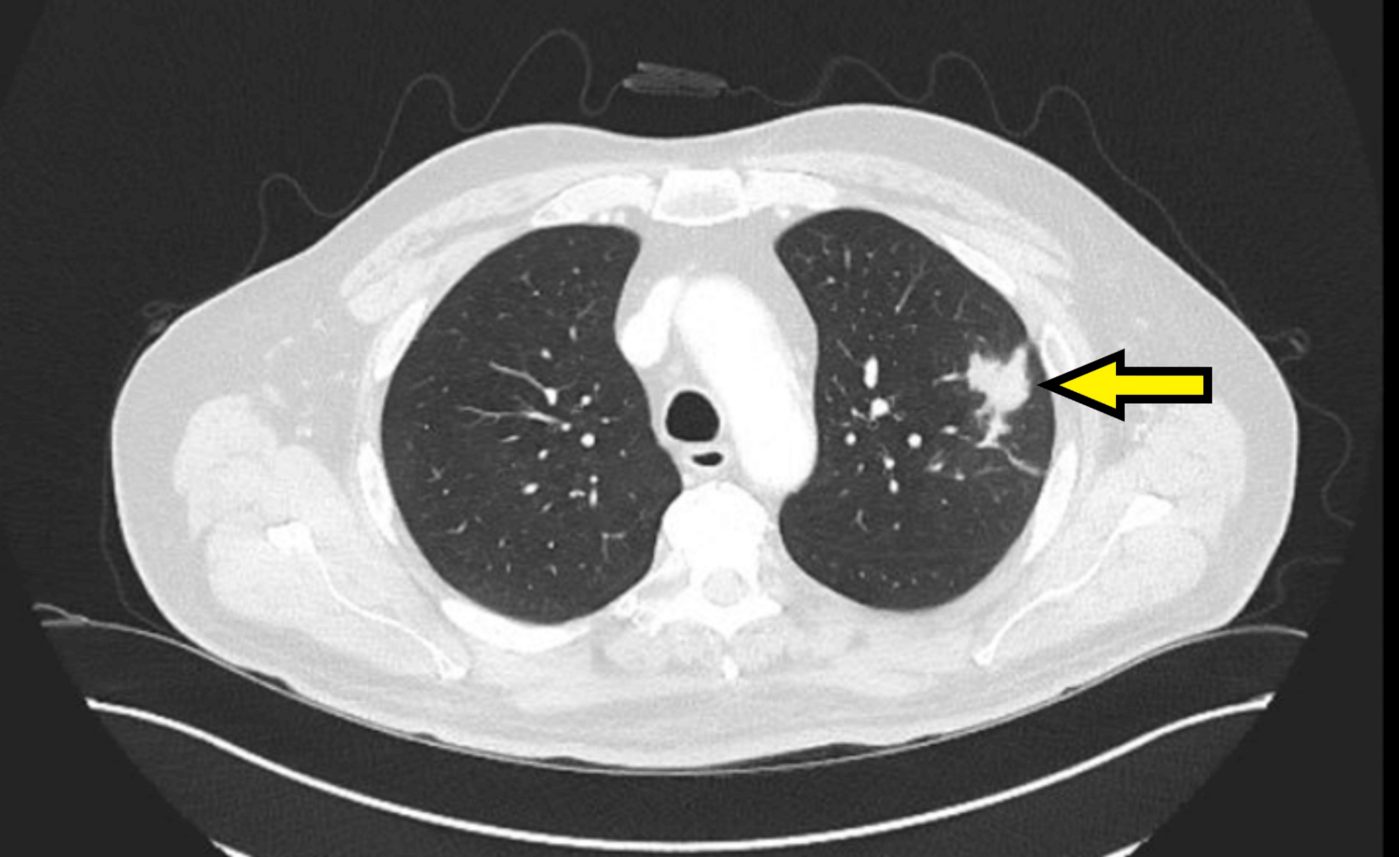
Axial CT scan of the chest demonstrating a 17 mm soft tissue nodule in the left upper lobe (yellow arrow) CT: computed tomography
